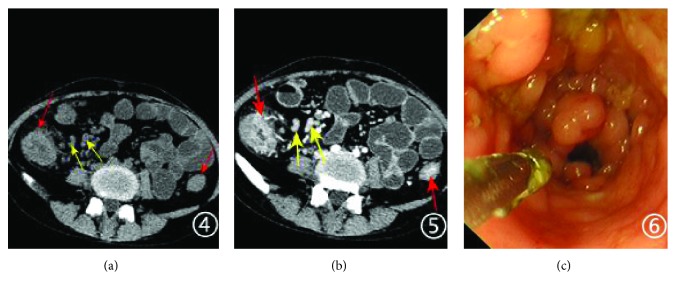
A 25-year-old women with mild active CD, SES-CD of 7. CT plain scan shows bowel wall thickening, peri-intestinal inflammation ((a) red arrowhead) in the ascending and descending colon, and mesenteric enlarged lymph nodes ((a) yellow arrowheads). Axial enhanced CT scan in the portal vein phase shows the thickened bowel wall of the ascending and descending colon and the stratified enhancement of thickened bowel wall ((b) red arrowheads) and mesenteric enlarged lymph nodes evenly enhanced ((b) yellow arrowheads). Endoscopic image is just like cobblestone (c).
